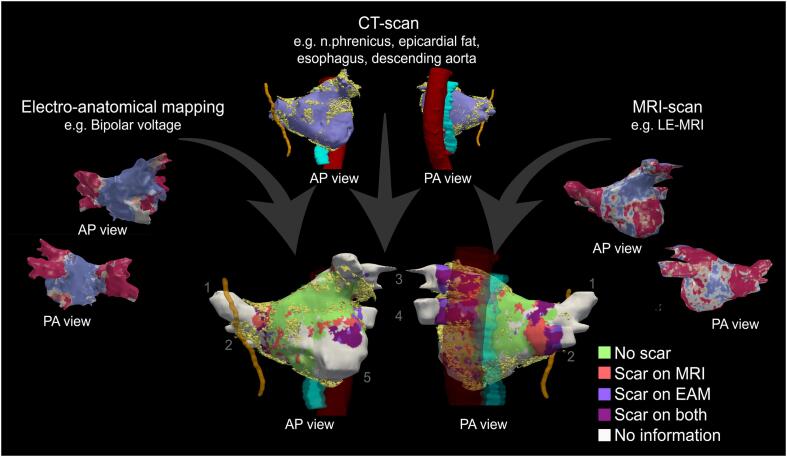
Example of a multi-modal re-do PVI roadmap. Alignment of different modalities allow for a multi-modal assessment of the left atrium and its relation to anatomical structures in its vicinity. In this example ‘scar’ was determined by low bipolar voltage in EAM and high signal intensity on LE-MRI. 1, Right superior PV; 2, Right inferior PV; 3, Left superior PV; 4, Left inferior PV; 5, Mitral valve anulus; AP view, anterior-posterior view; PA view, posterior-anterior view; CT, computed tomography; MRI, magnetic resonance imaging; LE, late gadolinium enhancement; EAM, electro-anatomical mapping.
